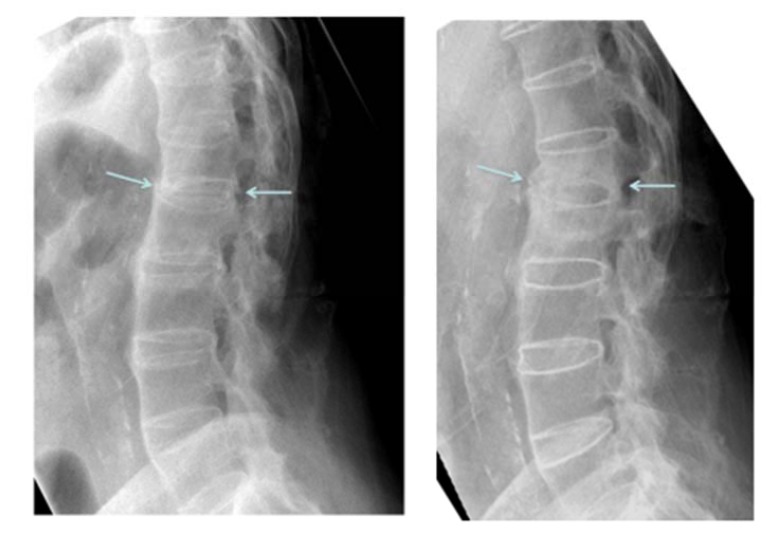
Arrows pointing to the anterior and middle column fracture areas on the initial lateral xray and follow up lateral xray.
